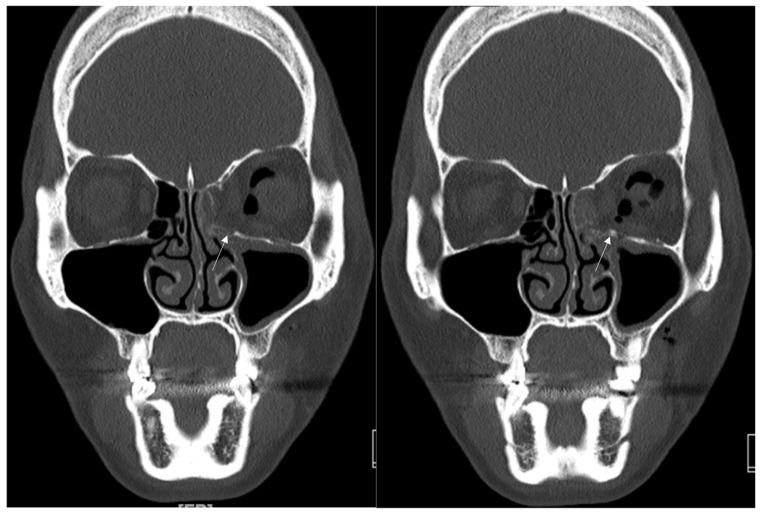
Case 2. Preoperative facial CT scan (coronal view) of the 48-year-old female patient with left inferomedial orbital wall fracture. The titanium-reinforced porous polyethylene (TR-PPE) plate was placed on the inferomedial orbital wall using a single screw at the inferior orbital rim. (White arrow).
